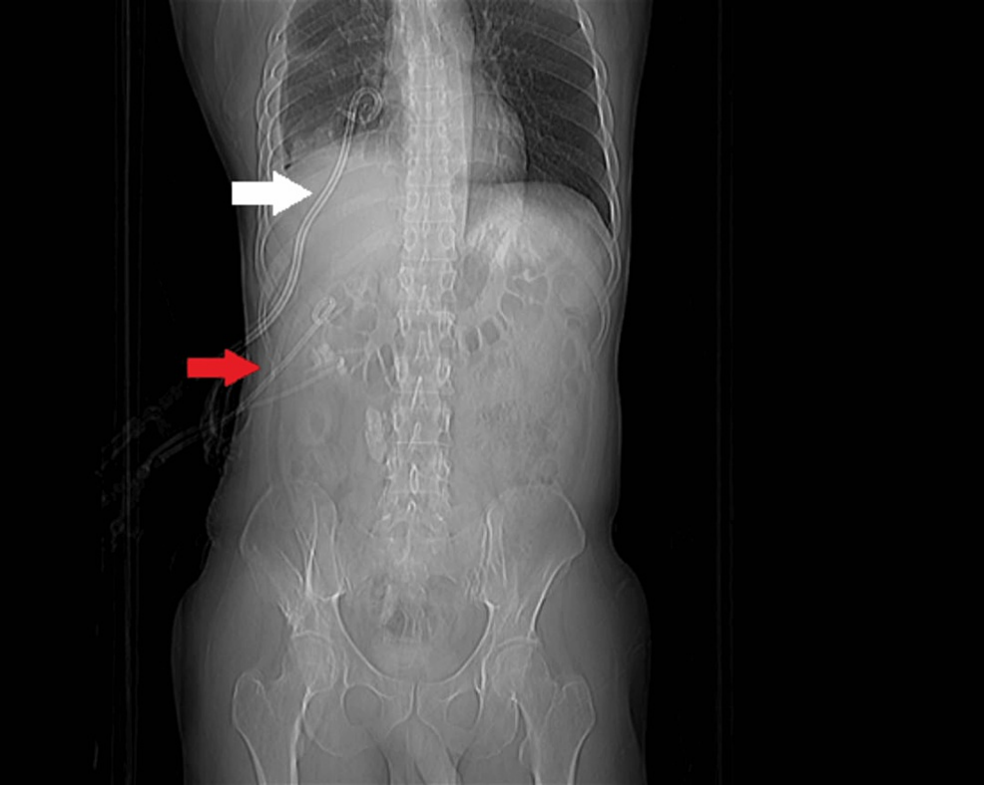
CT topogram chest and abdomen showing right chest tube (white arrow) and right renal drain (red arrow)
